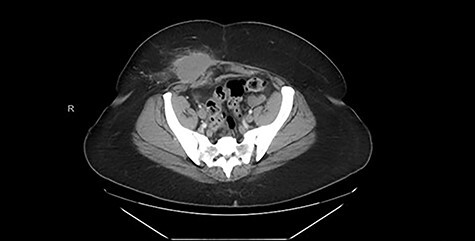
CT (axial view) demonstrating high-density soft tissue opacity measuring 5.8 × 4.4 cm in the subcutaneous tissue of the right lower quadrant abutting the anterior aspect of the abdominal wall.
